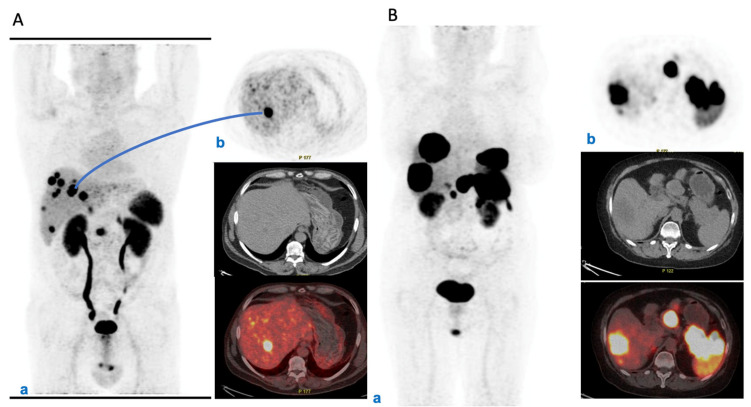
(A) PET/CT initial staging in metastatic pNET. (a) Male, 62 years old, pNEN, G1, initial staging. PET/CT ^68^Ga-DOTATOC MIP: depicts the intense uptake within primary pancreatic NEN (SUVmax 16.6) and in multiple liver metastases (SUVmax range: 6.6-62). (b) Axial image of the hottest liver metastasis along with corresponding CT and fused slice. (B) Female, 68 years old, pNEN, G3, staging during therapy with SSA and FOLFIRI. PET/CT ^68^Ga-DOTATOC MIP: (a) depicts the intense uptake within primary pancreatic NEN (SUVmax 38.6) and in large liver metastases (SUVmax range: 3-92). (b) Axial image of the primary pancreatic NEN, mesenteric lymph node, and largest liver metastasis.
